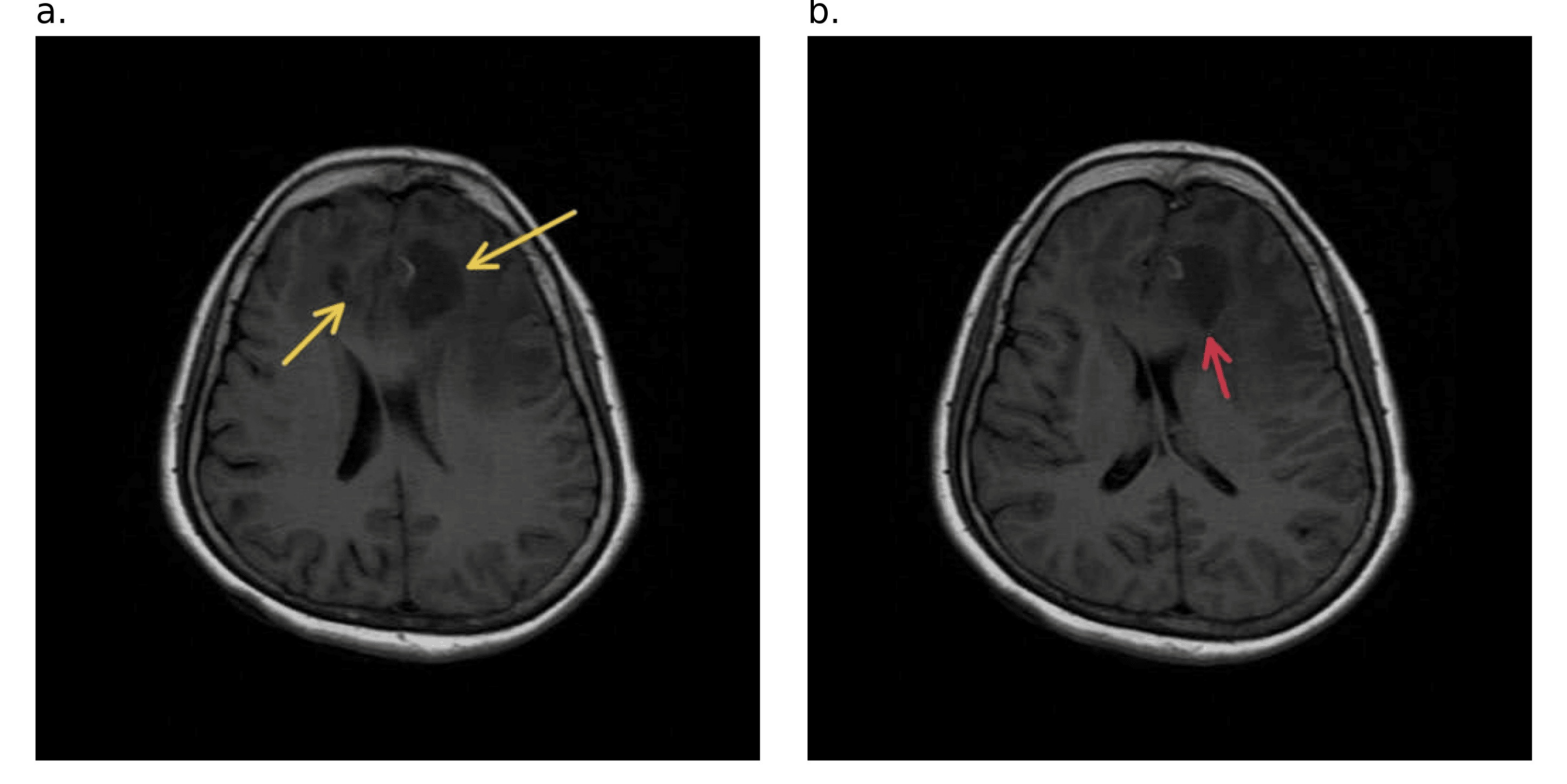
Axial T1-weighted MRI image a. The images show the peripherally enhancing tumor (yellow arrows) in the left frontal lobe at the level of the body of the lateral ventricles, and b. (red arrow) at the level of the capsuloganglionic region MRI: magnetic resonance imaging
